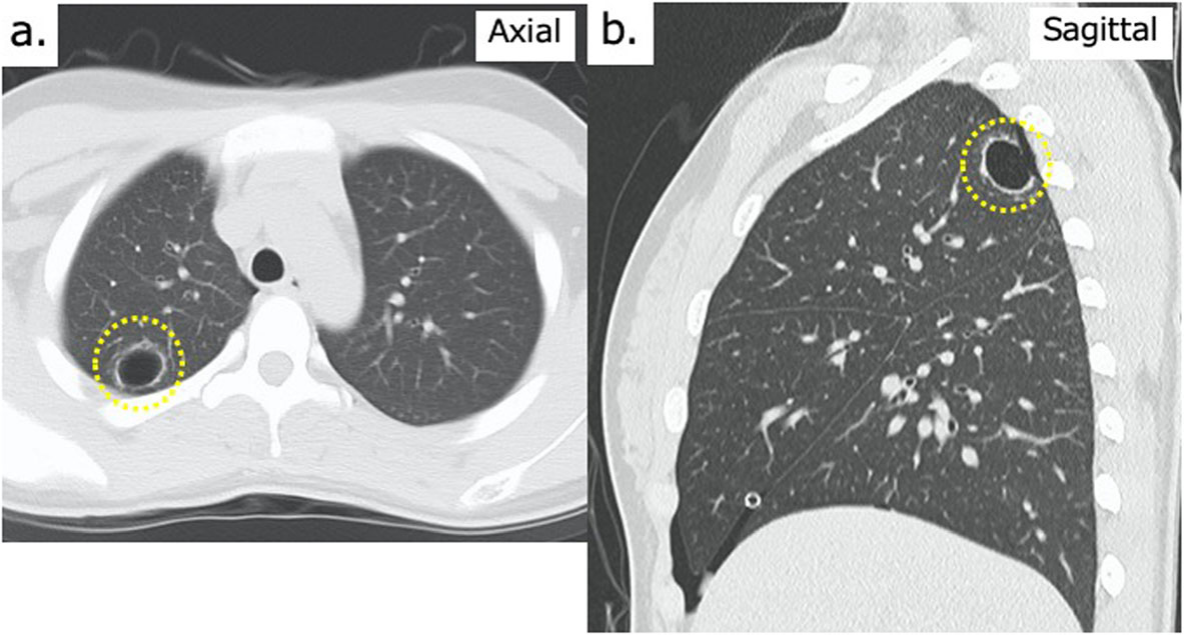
Preoperative chest computed tomography images. a Axial view showing a 19 × 17-cm cystic lesion in the right upper lobe. Thickening of the cyst wall is visible (dashed yellow circle). b Sagittal view showing a cyst located posterior to the apex of the right lung (S1-2)
